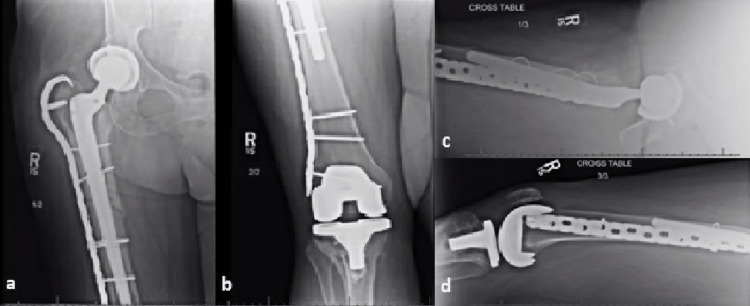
Three-month follow-up radiographs of the right hip including proximal femur AP (a), distal femur AP (b), proximal lateral (c), and distal lateral (d) views. Radiographs do not show any evidence of subsidence and the fracture remains stable.
